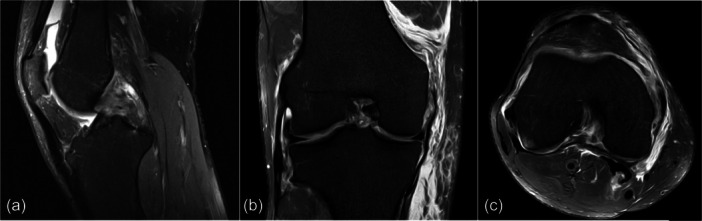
Exemplary representation of T2‐weighted MRI images depicting: (a) typical case of an acutely ruptured posterior cruciate ligament on sagittal view, (b) III femoral rupture of superficial medial collateral ligament on coronal view and (c) rupture of posterior oblique ligament on axial view. MRI, magnetic resonance imaging.
